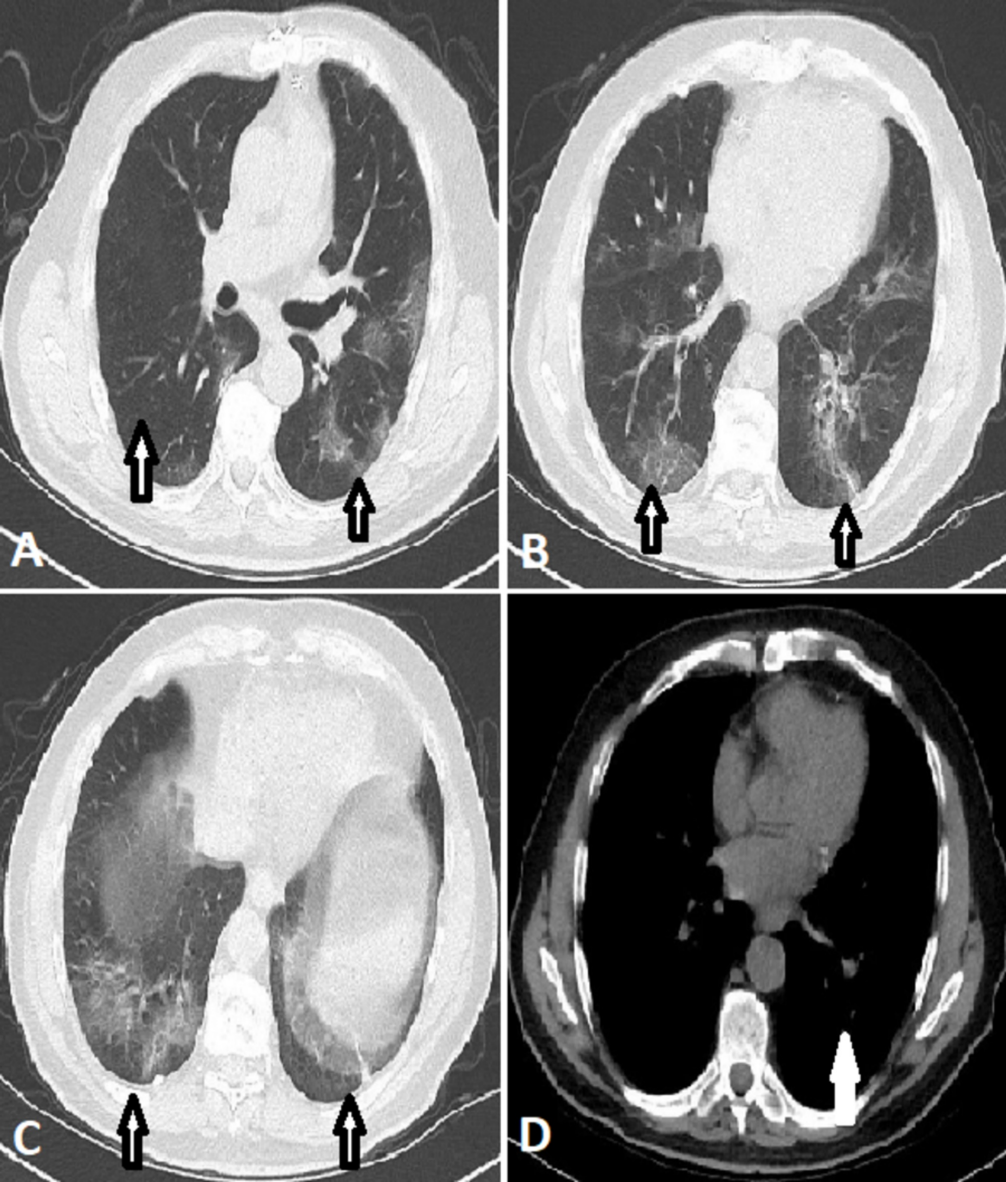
CT scan of chest These are axial views of CT scan of the chest showing multiple ground-glass opacities in the left middle and both lower zones in the peripheral distribution
